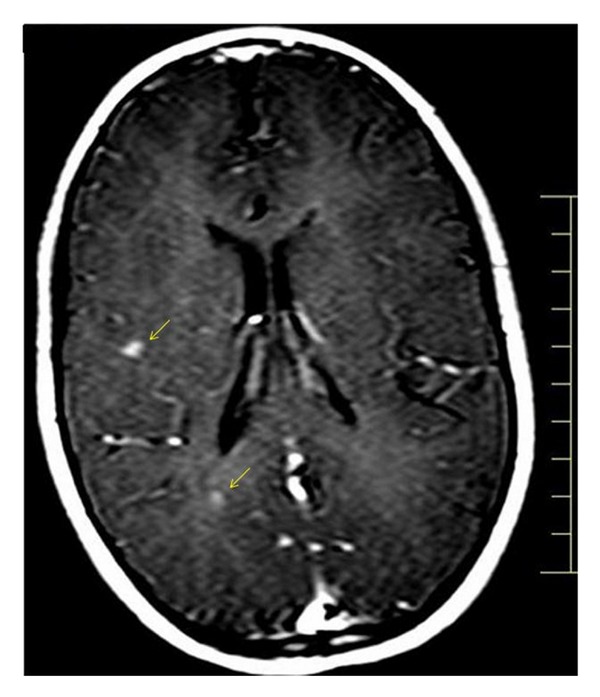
Brain MRI showing multiple gadolinium-enhancing lesions (arrows).
